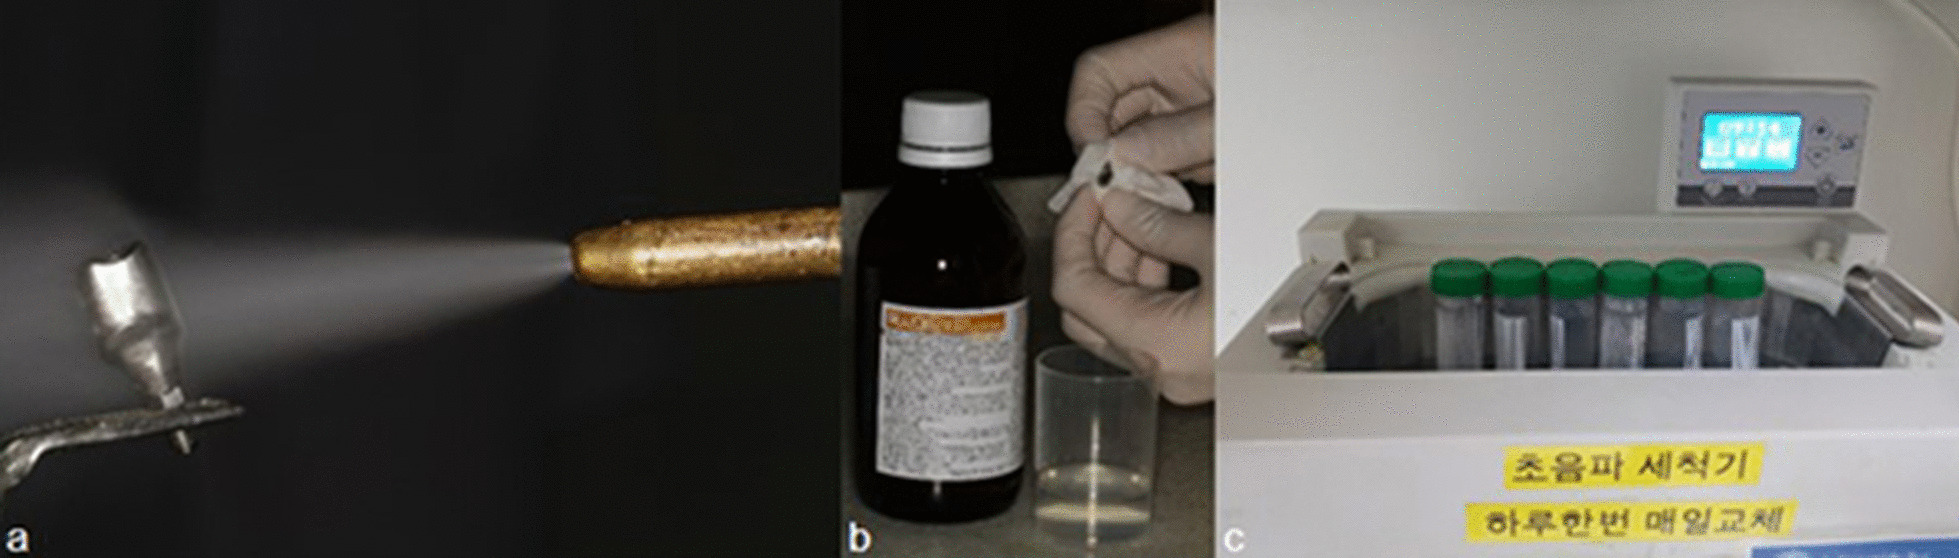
Customized titanium abutments cleaned with three different methods: a steam cleaning, b CHX scrubbing, and c ultrasonic cleaning
